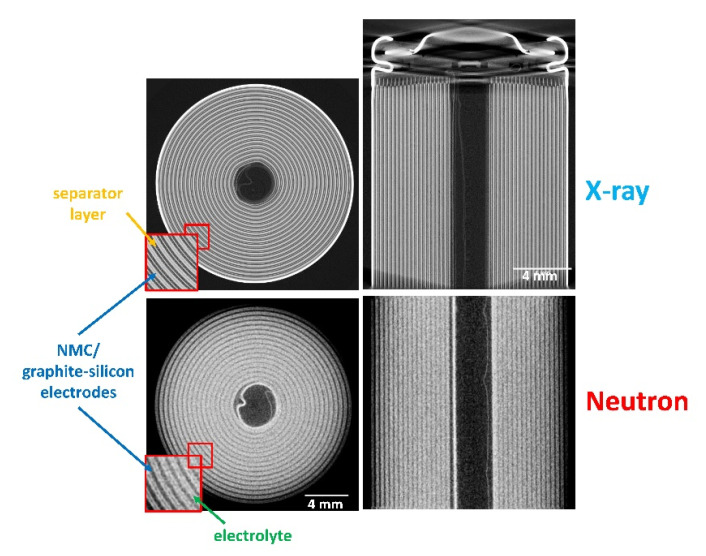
Comparison of X-ray and high-resolution neutron tomography with the same pixel size (6.5 µm) of a Li-ion cell (LG 18650 MJ1) with NMC cathode and new graphite-silicon anode for a high capacity of 3500 mAh. Image courtesy: Ralf Ziesche, UCL, UK.
